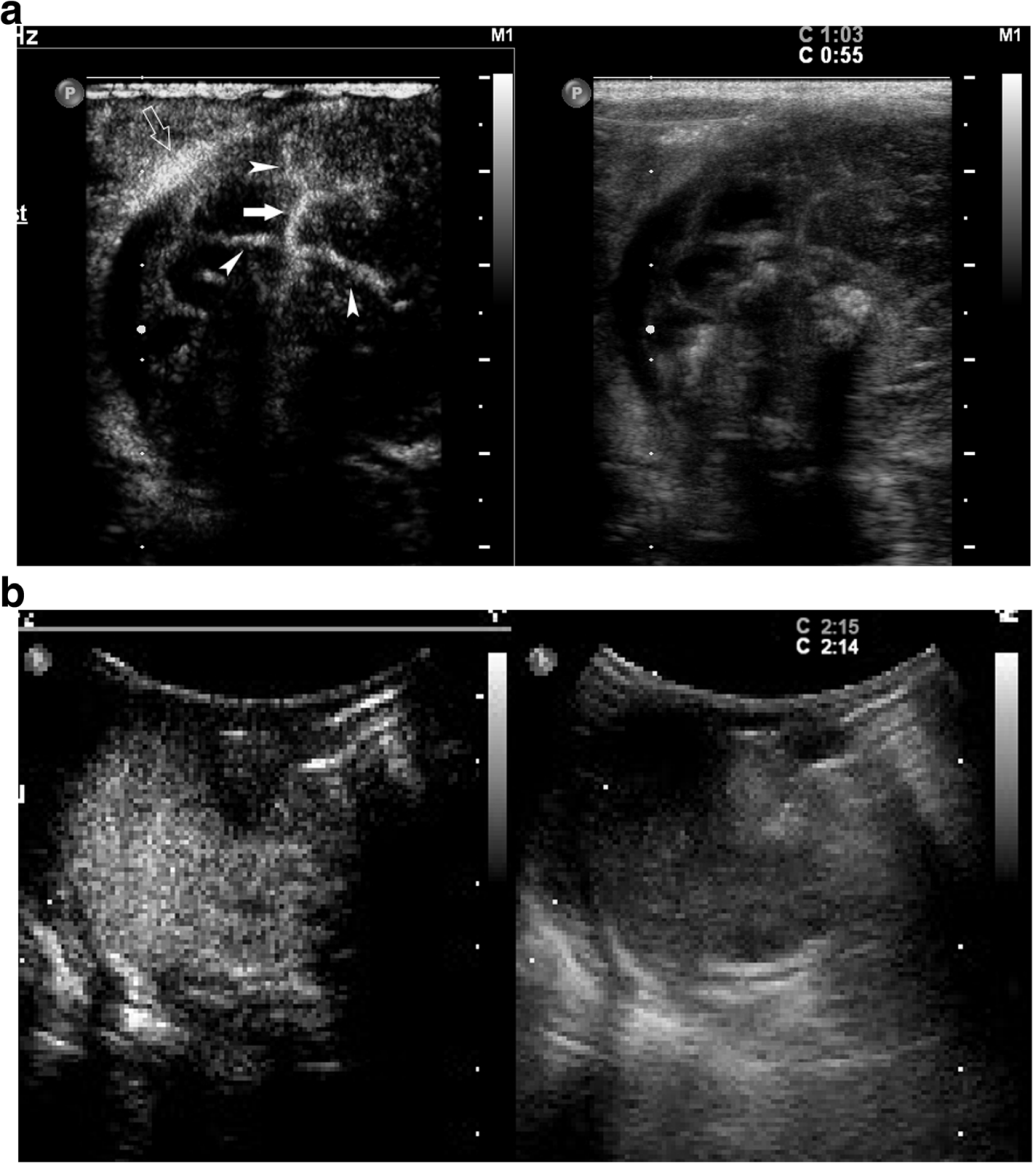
Contrast-enhanced ultrasound (CEUS) imaging (left) and corresponding grayscale ultrasound imaging (right): CEUS clearly showing that the abdominal aorta-like structure (white arrow), limb branch vessel-like structure (white arrowhead), and umbilical vessel-like structure (hollow arrow) in the mass are enhanced at 55 s (a), and the mass is persistently enhanced until 2 min and 14 s (b)
